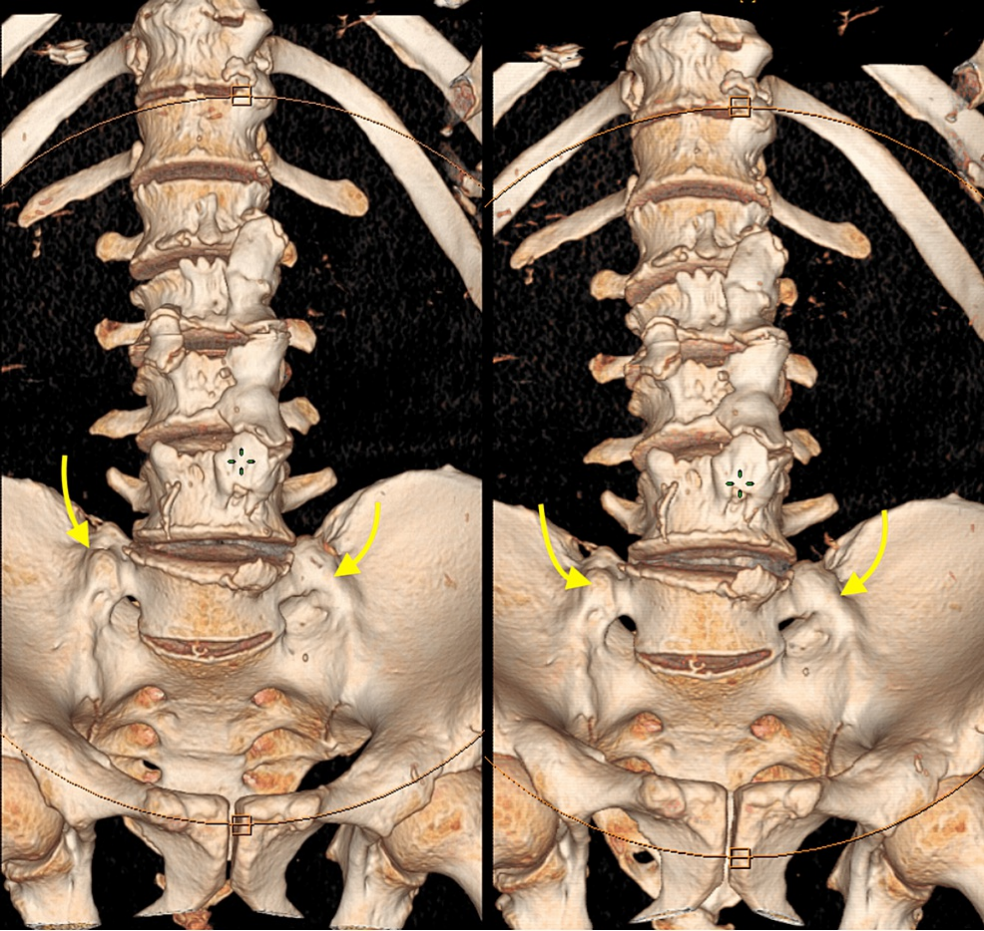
3D reconstruction of lumbosacral spine CT showing bilateral transverse mega-apophysis of L5 (yellow arrows) articulating with the sacrum and ilium. 3D: three-dimensional, CT: computed tomography
